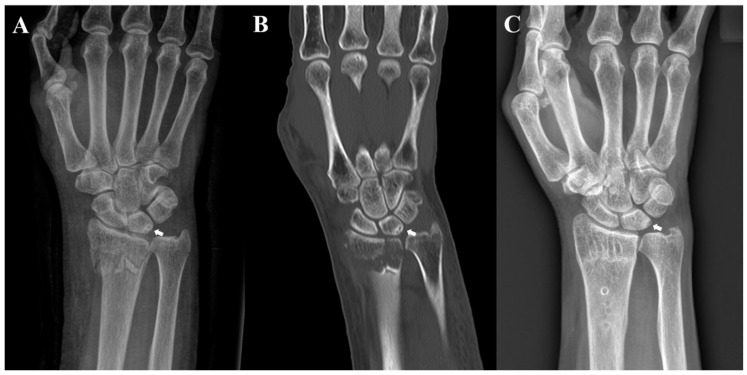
Radiographs of a 70-year-old woman. (A) Preoperative posteroanterior radiograph and (B) coronal computed tomography image revealing sclerosis with cystic changes on the ulnar side of the lunate; (C) posteroanterior radiograph acquired after the removal of internal fixation at 12 months after surgery reveals the remnant sclerotic lesion with cystic change of the lunate.
